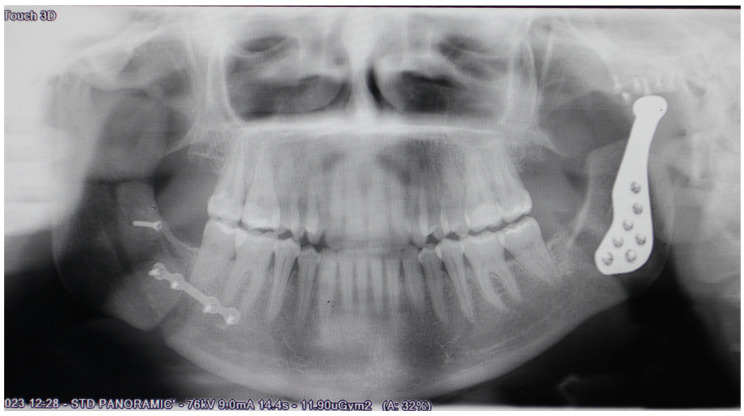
Follow-up orthopantomography (6 months) showing left total joint prostheses in place.
